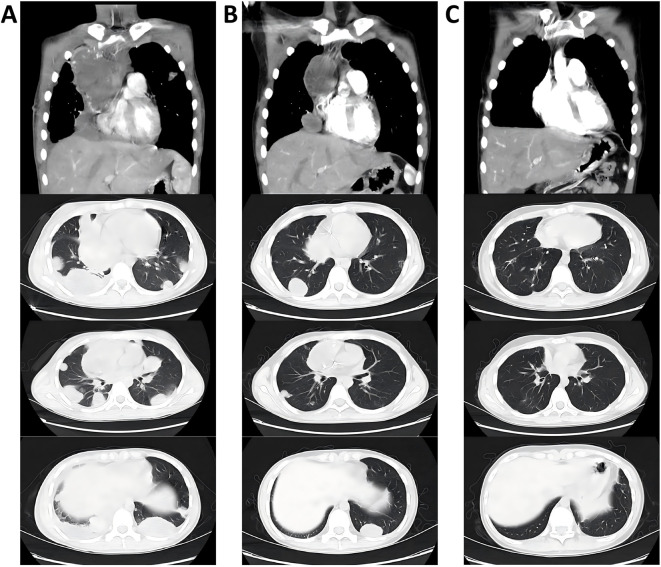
Dynamic CT evaluation of mediastinal mass and pulmonary lesions. (A) at diagnosis. (B) after six cycles of NACT. (C) five months after the end of treatment.
